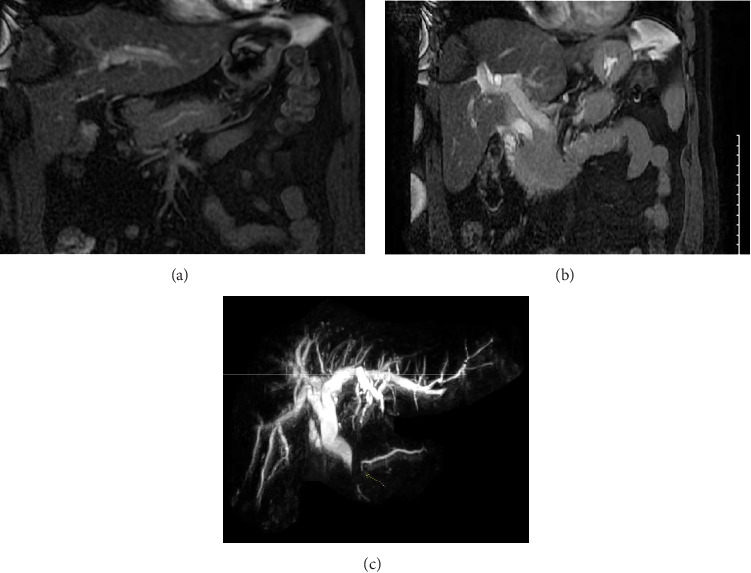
MRI abdomen with and without contrast (a). Focal enhancement along the medial aspect of the distal CBD (b). Abrupt termination of the pancreatic duct signal (c).
